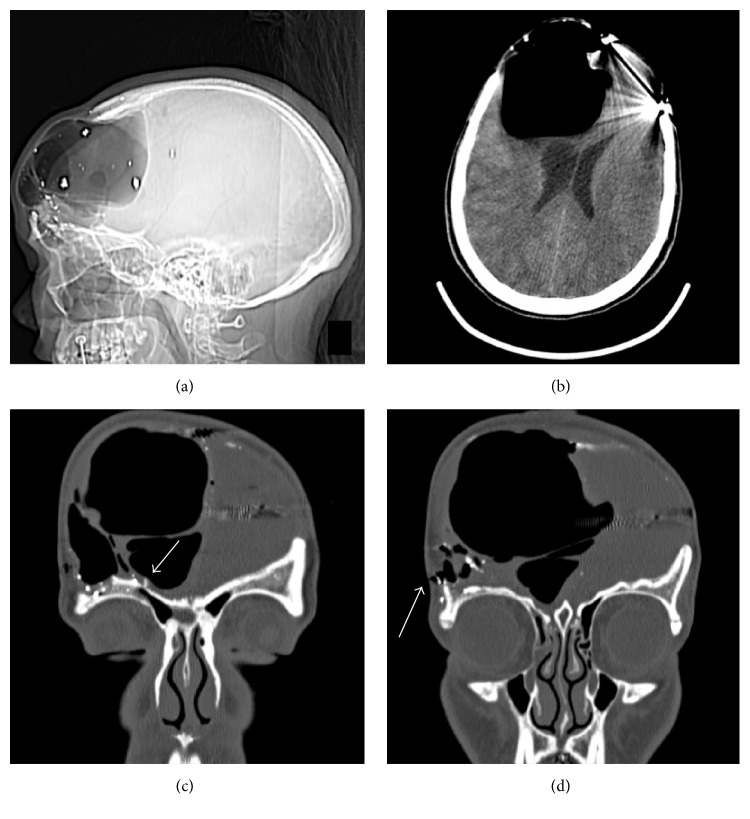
Readmission CT head and maxillofacial-sinus scan. (a) Scout image demonstrating a large pocket of hypodensity in the right frontal region consistent with pneumocephalus. (b) Axial CT demonstrating right frontal tension pneumocephalus with significant mass effect including effacement of the right lateral ventricle, midline shift, and subfalcine herniation. (c, d) Coronal reconstructed images demonstrating air communicating through the skin in the right orbital-frontal region and a small defect with shard of bone in the superior aspect of the right frontal sinus.
